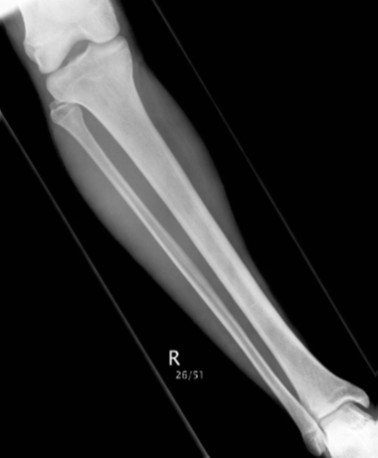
AP X-ray shows tibia and fibula.
